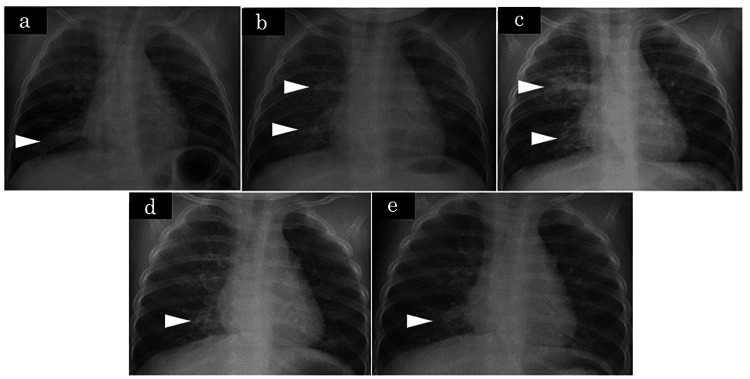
Chest XP Chest XP at (a) Month 6, (b) Month 7, (c) Month 8, after bronchoscopy, (d) Month 10, and (e) Month 11. ▷ shows an image of pneumonia. XP: X-ray photography.
